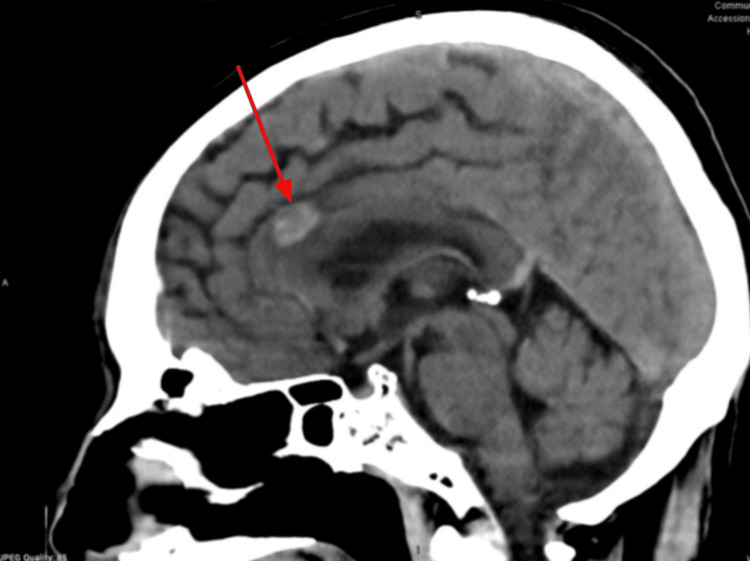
Brain MRI (June 2024) showing stability of metastases
